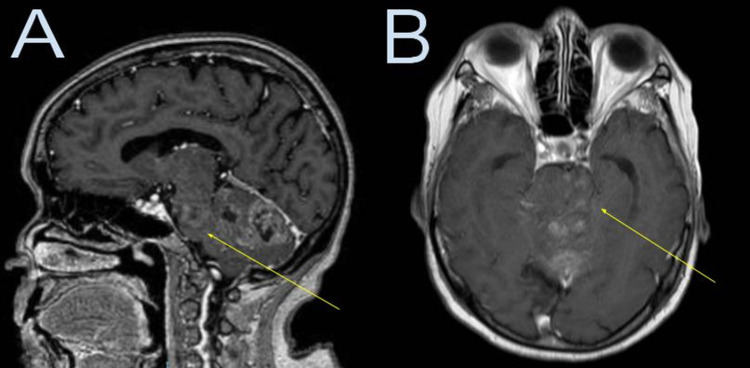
Brain MRI with contrast 30 days later Brain MRI with contrast 30 days later (A: sagittal, B: axial) shows an enlargement of the cerebellar mass, now extending to the brainstem (yellow arrow) with infiltration of the left brachium pontis, the left posterior aspect of the tegmentum of the pons, and posterolateral medulla oblongata.
